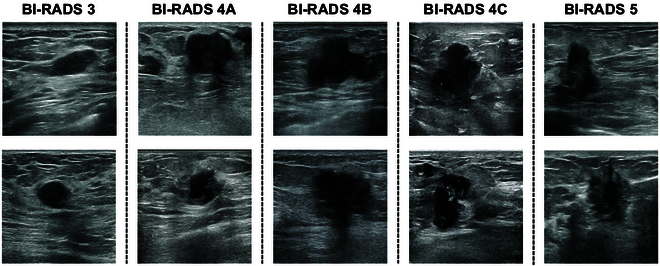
Examples of CoLDiT-generated breast ultrasound (US) images featuring different BI-RADS categories (including BI-RADS 3, 4A, 4B, 4C, and 5).
